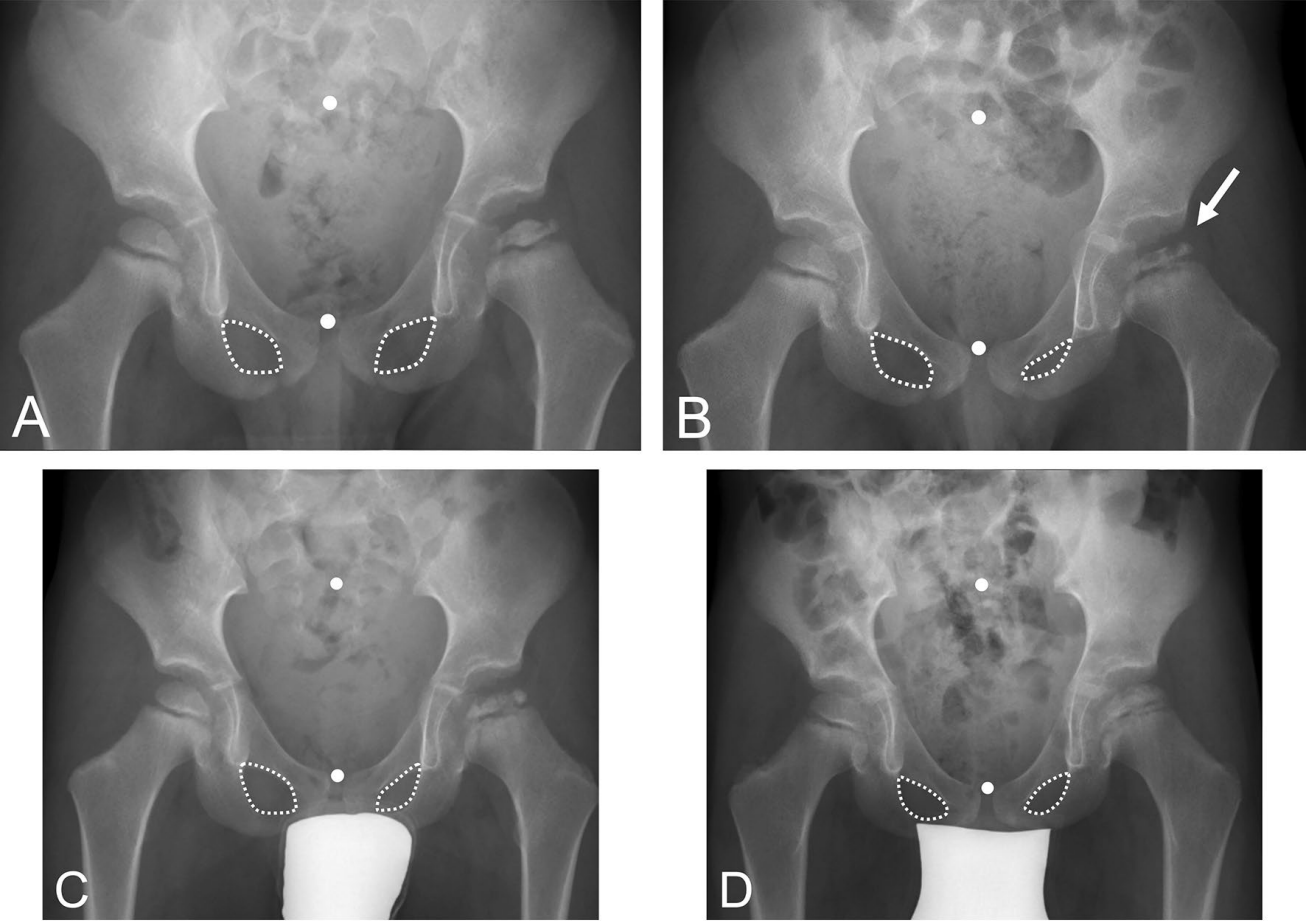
Four-year old boy with Legg–Calvé–Perthes Disease (LCPD) on the left hip at A the initial stage (Waldenström I [24]) showing a symmetrical shape of the obturator foramen. B At the fragmentation stage (arrow, Waldenström II [24]), an asymmetrical shape of the obturator foramen indicating acetabular retroversion can be seen despite correct pelvic position during radiograph acquisition. C With progressive healing of LCPD the foramen become more symmetrical again (Waldenström III [24]) but D some asymmetry remains in the healed stage (Waldenström IV [24]). The white dots (Fig. 6A–D) indicate the mid of the sacrum and symphysis indicating correct pelvic rotation and constant pelvic tilt during radiograph acquisition
